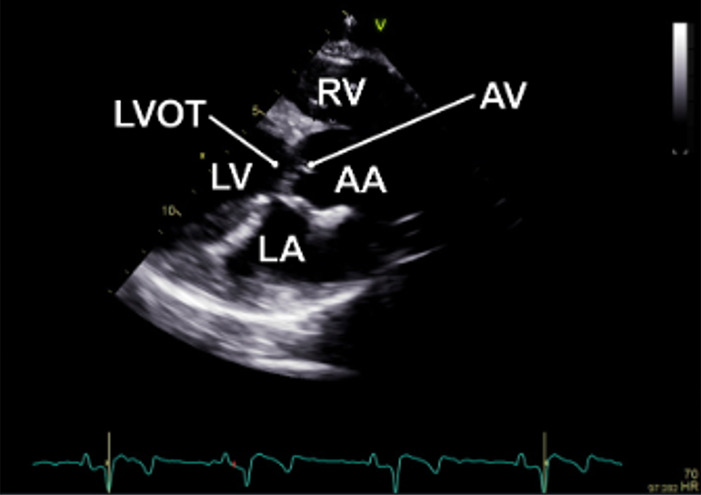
• Transducer left parasternal position	• LV • LA • LVOT • AV • RV
